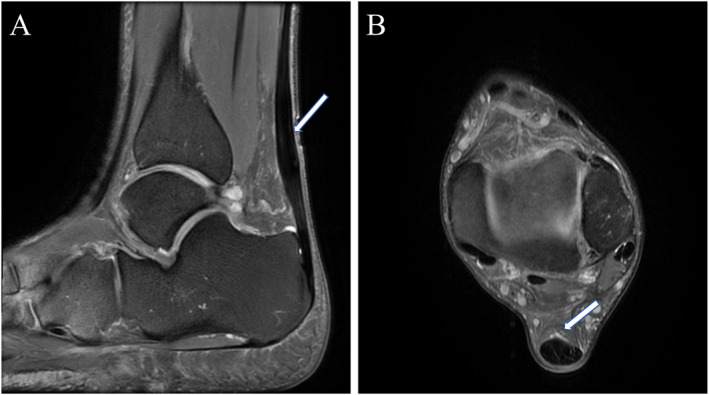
A 50‐year‐old male amateur marathon runner with a 7‐year running experience and a monthly running distance of 200 km; representative MRI of midsection Achilles tendinopathy. (A) Sagittal fs‐PDWI of the left ankle shows fusiform expansion of the mid‐Achilles tendon with linear hyperintense signals (arrow) and (B) axial fs‐PDWI of the left ankle demonstrates thickening of the Achilles tendon, increased anteroposterior diameter, and heightened internal signal (arrow).
